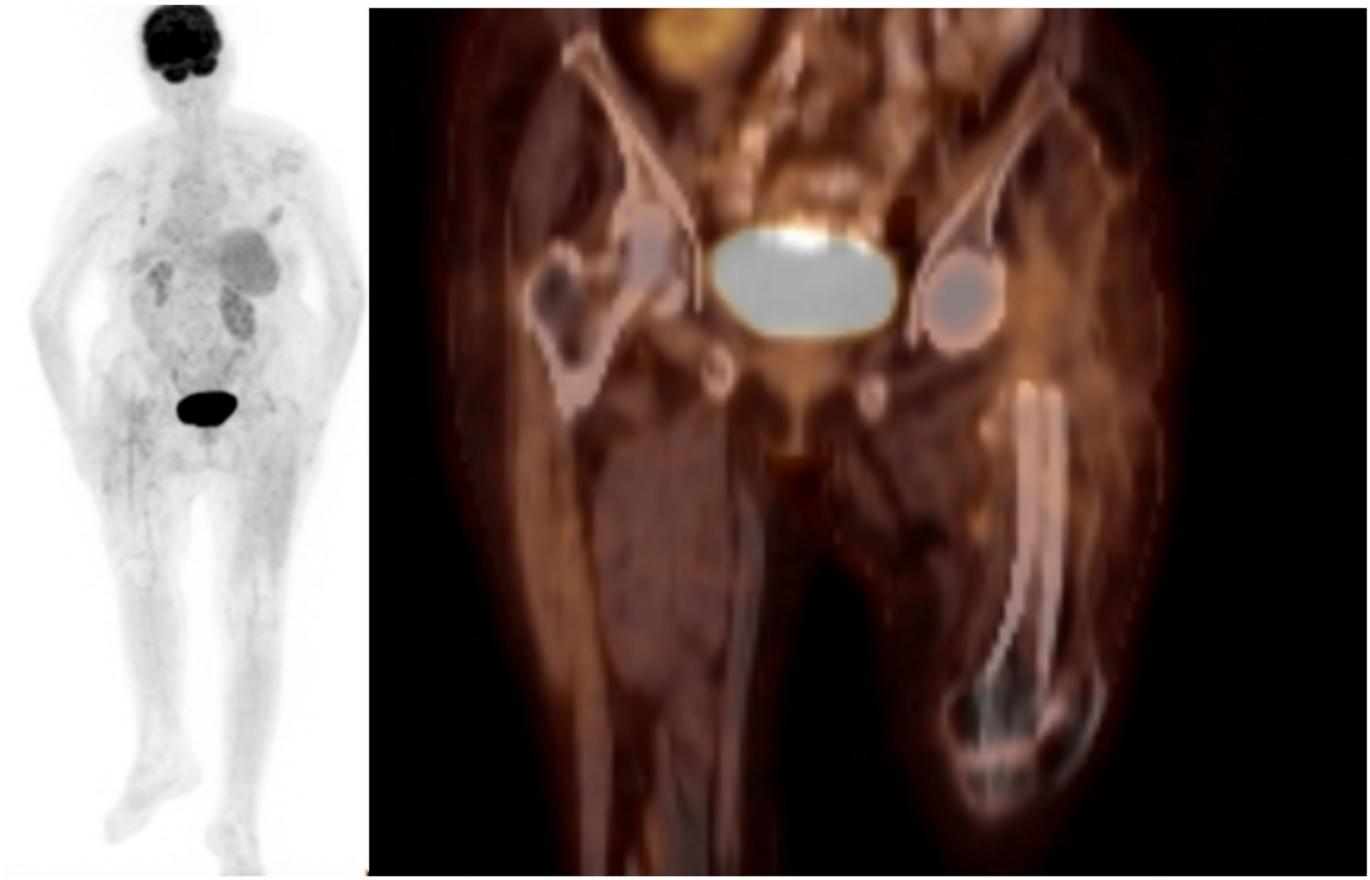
(Negative example) Representative 18F-FDG PET/CT images demonstrating diffuse, homogeneous periarticular FDG uptake without focal activity at the bone–cement interface, classified as non-suggestive of infection according to the predefined criteria. (SUV-max: 2.7)
